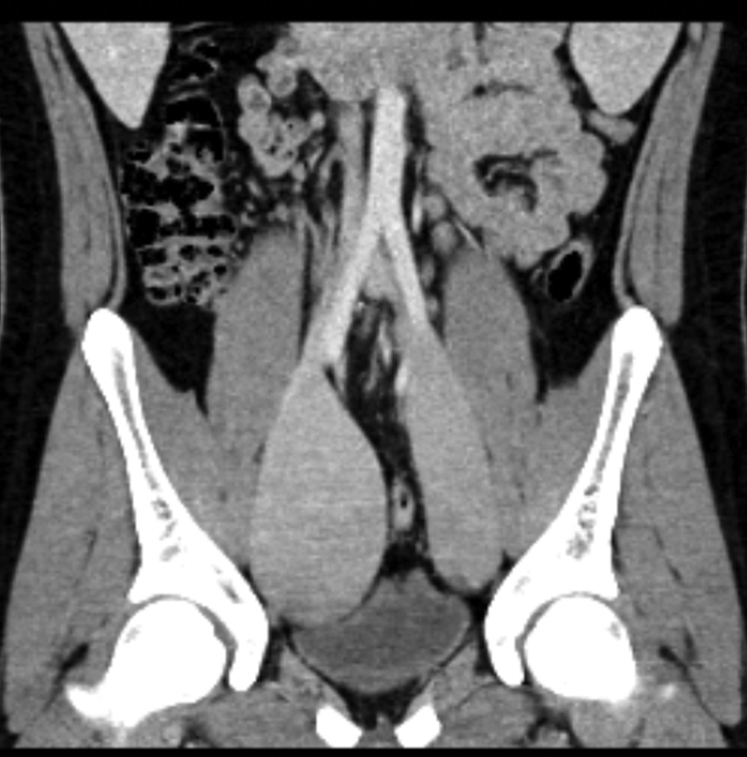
Coronal computed tomography image showing bilateral external iliac vein aneurysms.
